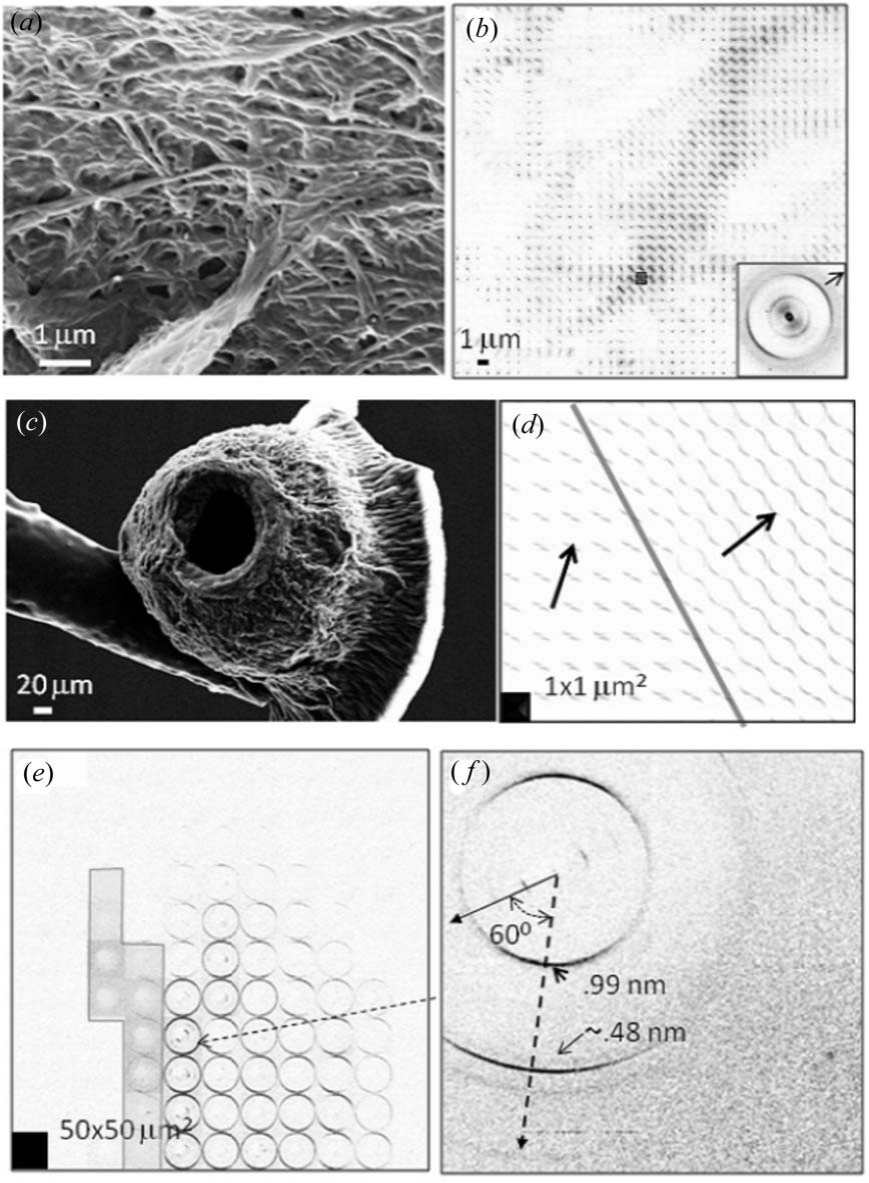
(*a*) FESEM image of fibrillar Ac-NFGAIL morphology (Lakshmanan *et al.*, 2013 ▶). (*b*) Raster-scan image of fibrillar morphology obtained with a 200 nm X-ray beam. The inset shows a single WAXS pattern from the position of the rectangle in (*b*). The fibre axis is indicated by an arrow. [Adapted from Lakshmanan *et al.* (2013 ▶).] (*c*) SEM image of hollow Ac-KLVFFAE residue glued to a glass capillary (Lakshmanan *et al.*, 2013 ▶). (*d*) Raster-scan image of bulk residue revealing two domains with homogeneous fibre orientation (arrow) in each domain defined by the orientation of the 0.47 nm β-sheet peak. (*e*) Raster-scan of residue glued to the capillary (contours indicated) with oriented patterns from the pinning zone at the hole [see also (*c*)]. (*f*) Oriented pattern with two principal reciprocal lattice lines at an angle of ∼60°. The first and second (weak) cross-β peaks are indicated. [Adapted from Lakshmanan *et al.* (2013 ▶).]
